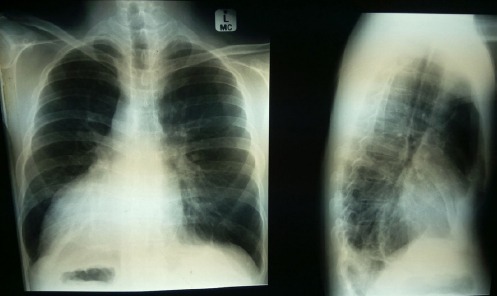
Chest X-ray showing a right-sided cardiac shadow along with cardiomegaly. Note the right-sided gastric bubble
